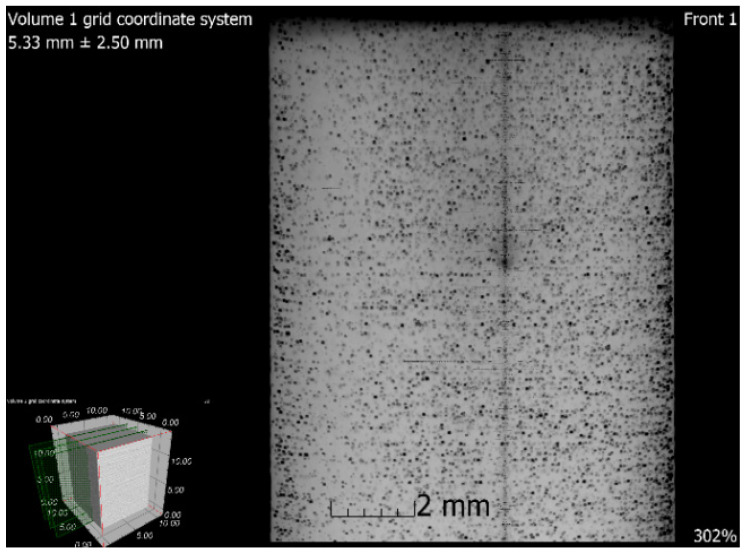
Tomographic CT sections of sample 5 after SLM (XZ plane).
